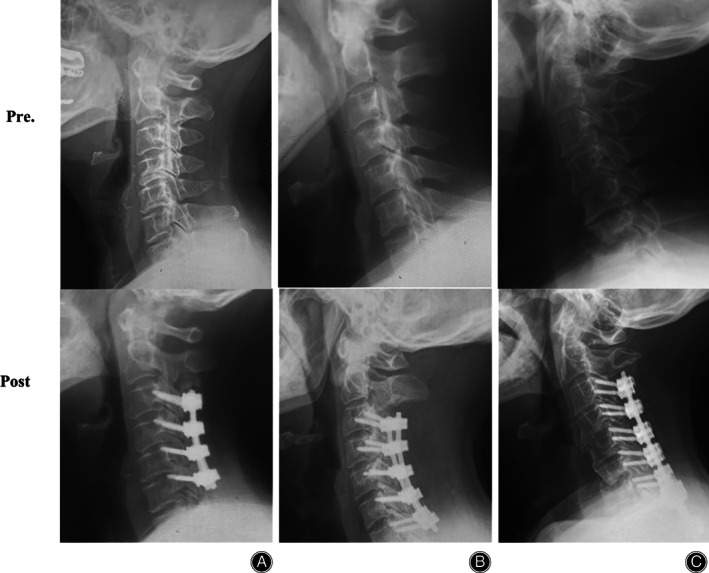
Representative images regarding the influence of different distal fusion level on radiographic outcomes. (A) Case 1, a 56‐year old male patient with continuous cervical OPLL from C_2_ to C_5_ was admitted to our institution. His preoperative NDI score was 36. Preoperative lateral X ray indicated that his cervical lordosis was 10.8° (A, upper). Laminectomy with instrumented fusion from C_3_ to C_6_ was given, with the distal fusion terminating at C_6_. At the final follow‐up, his NDI score improved to 14. And his cervical lordosis was 14.9°, with the change of Cobb angle of 4.1° (A, nether). (B) Case 2, a 53‐year old male patient with continuous cervical segmental OPLL from C_4_ to _C6_ was admitted to our institution. His preoperative NDI score was 37. Preoperative lateral X ray indicated that his cervical lordosis was 7.5° (B, upper). Laminectomy with instrumented fusion from C_3_ to C_6_ was given, with the distal fusion terminating at C_7_. At the final follow‐up, his NDI score improved to 16. And his cervical lordosis was 10.6°, with the change of Cobb angle of 3.1° (B, nether). (C) Case 3, a 46‐year old male patient with continuous cervical OPLL from C_3_ to C_7_ was admitted to our institution. His preoperative NDI score was 38. Preoperative lateral X ray indicated that his cervical lordosis was 11.9° (B, upper). Laminectomy with instrumented fusion from C_3_ to C_6_ was given, with the distal fusion terminating at T_1_. At the final follow‐up, his NDI score improved to 17. And his cervical lordosis was 10.4°, with the change of Cobb angle of −1.5° (B, nether).
